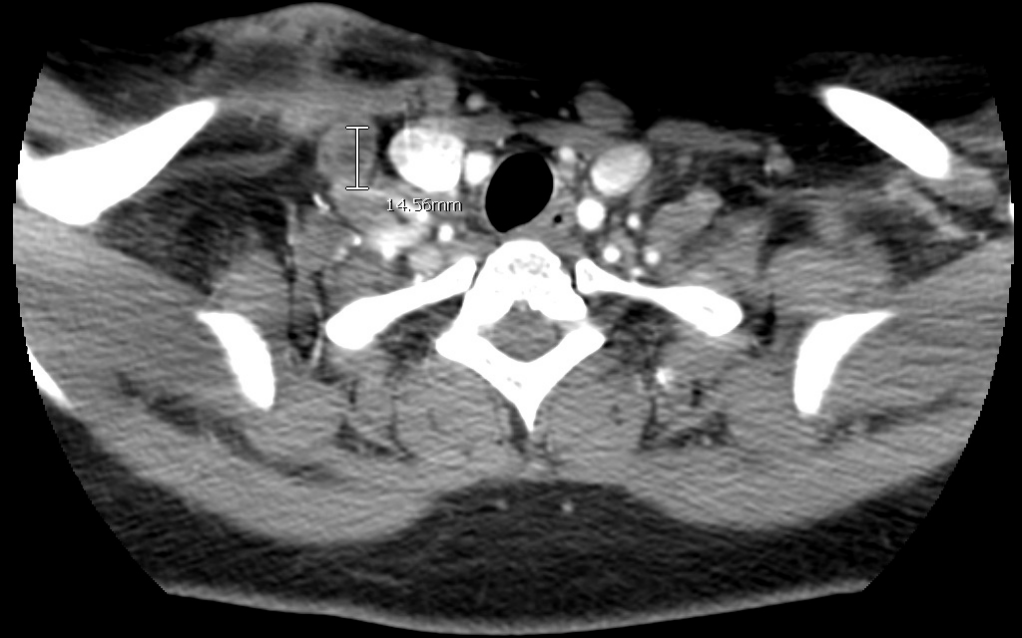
Computed tomography scan of the neck with supraclavicular lymph node
